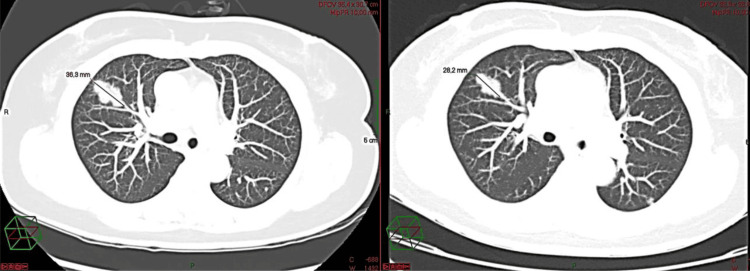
Follow-up HRCT 10 months later revealing interval growth of the right upper lobe lesion to 36 mm
